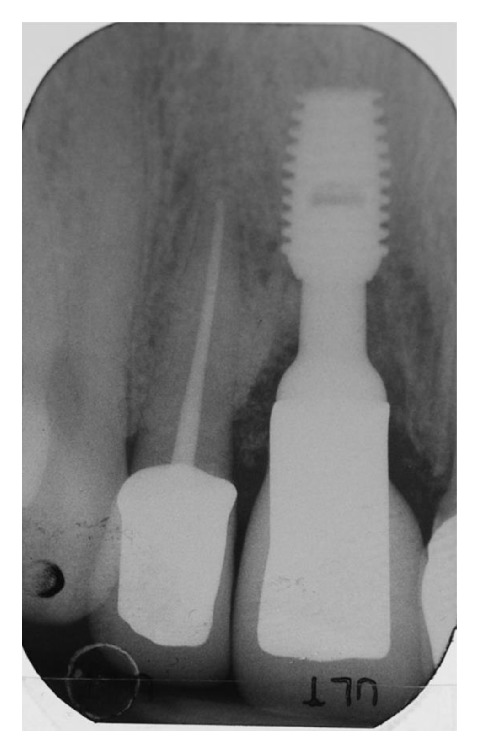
The radiograph performed after five years shows full integration of the implant and good stability of interproximal soft tissues, along with almost full filling of the lateral incisor's periodontal defect.
